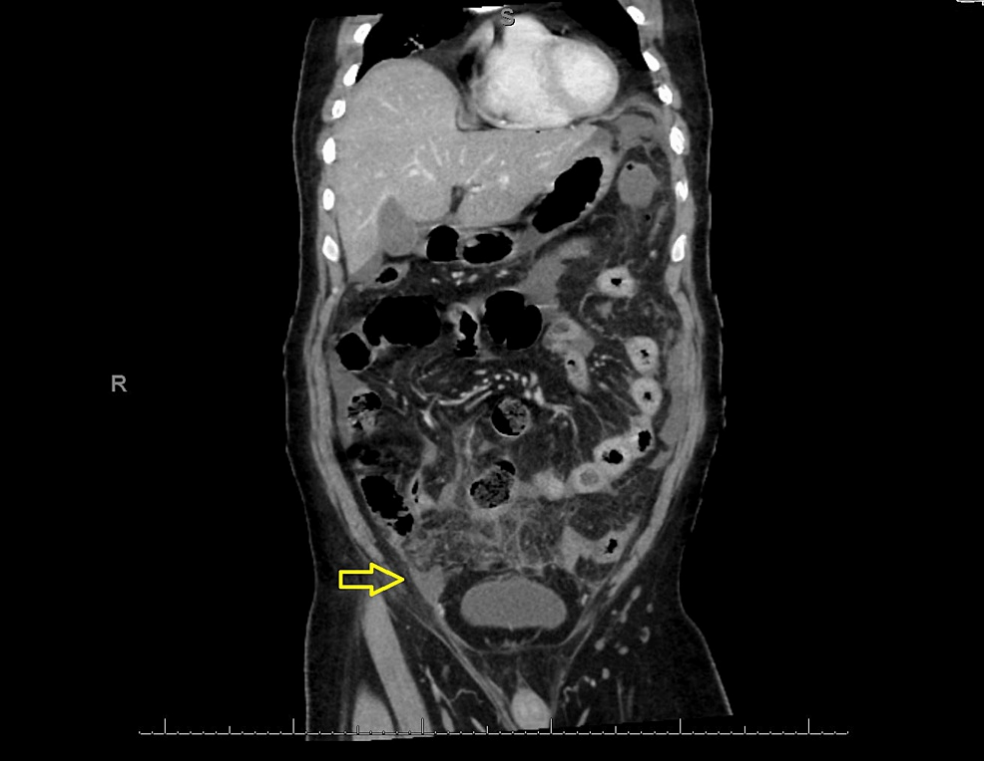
The CT scan shows findings of perforated appendicitis. The area of pus collection is labeled with an arrow on the right, and there is no extension of pus collection into the right inguinal canal.
